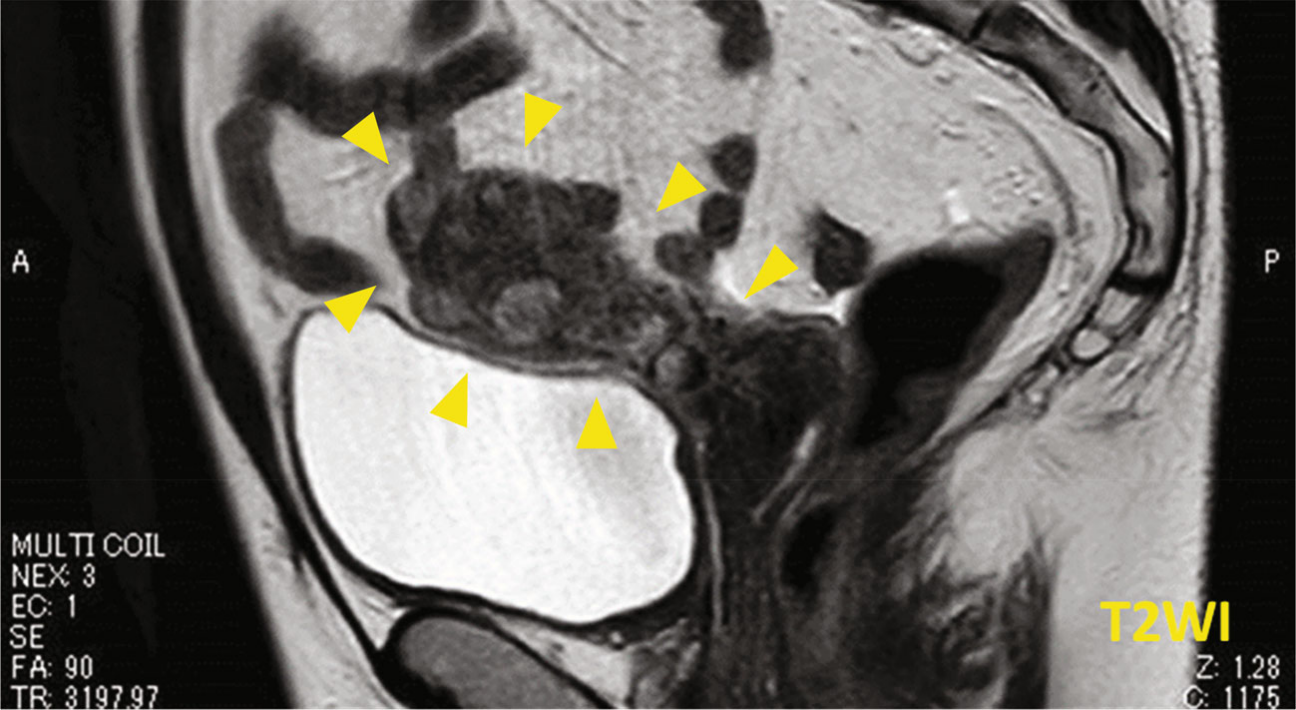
Magnetic resonance imaging (MRI) revealed multiple masses extending from the posterior wall of the uterus to the basal layer of the myometrium.
